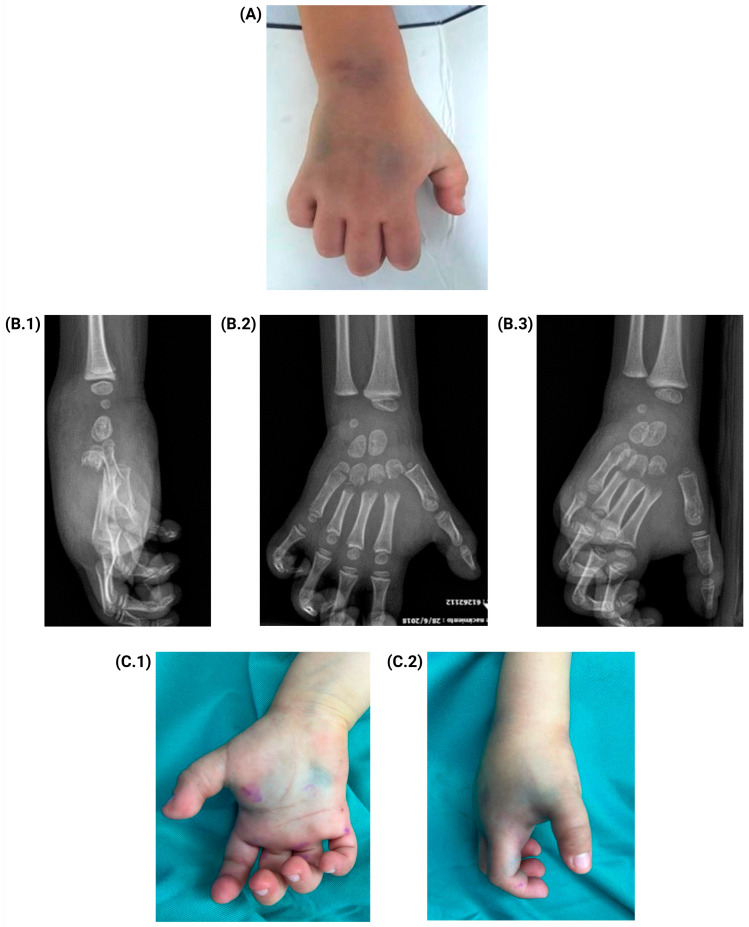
Condition of the patient’s hand on arrival. (A) Physical examination of the hand upon arrival at the emergency room. (B.1–B.3) Lateral, anteroposterior, and posteroanterior radiographs of the hand. (C.1,C.2) Condition of the patient’s hand prior to resolutive surgery.
